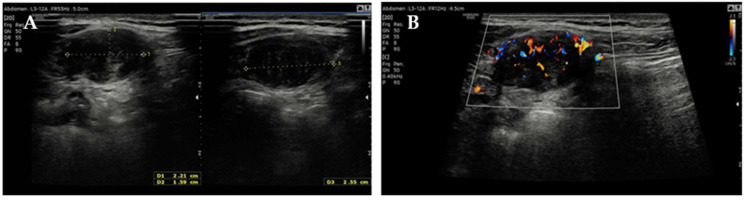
Transabdominal US of the right abdominal wall: (A) measurements of the lesion and (B) its color-Doppler vascularization.
